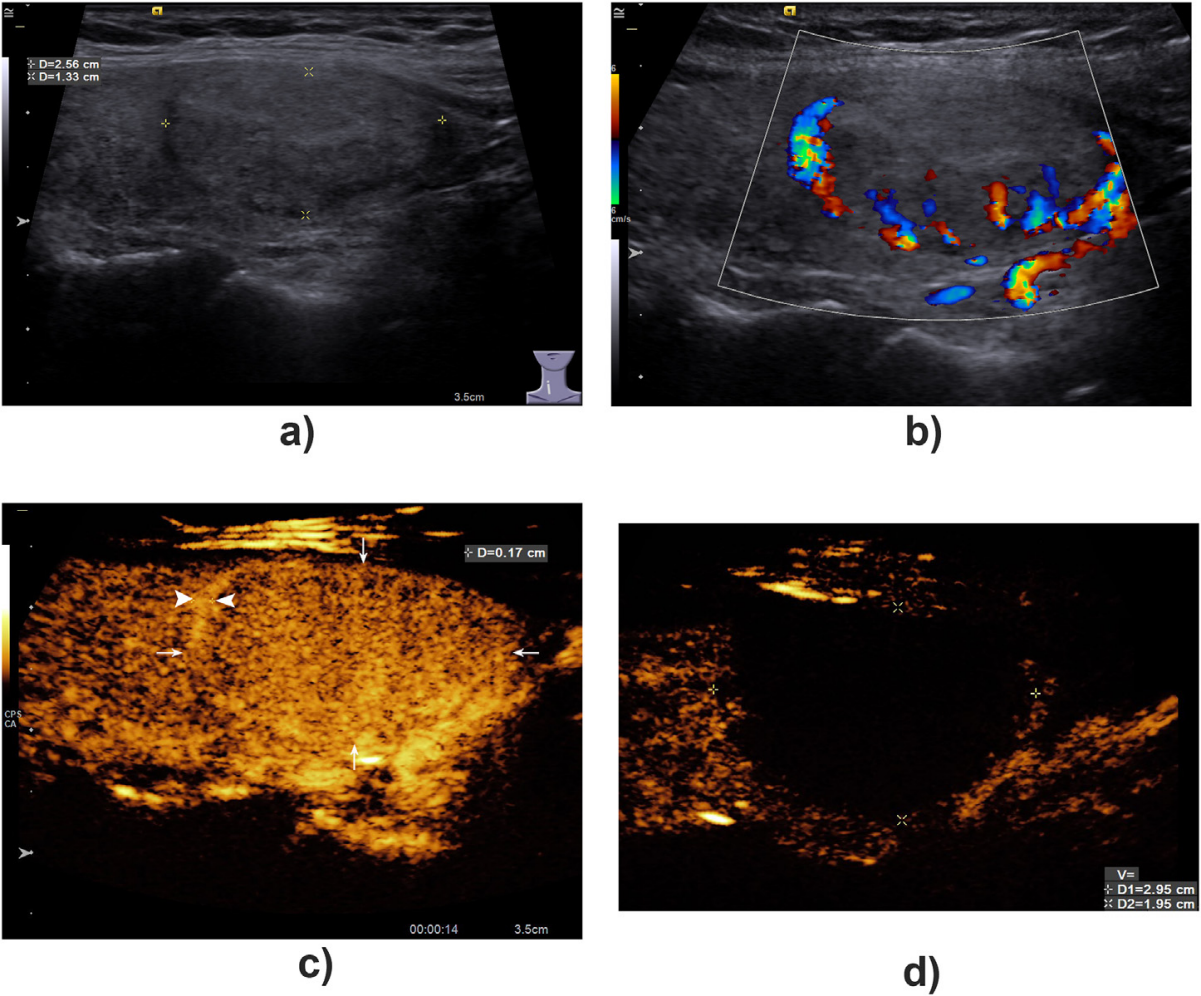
A BTN with a hyper-enhancement ring on CEUS. A 36-year-old woman with a benign nodule in her right lobe of thyroid for 8 years follow-up. The size of the nodule was 2.56 × 1.33 cm before ablation treatment. Conventional ultrasound showed that the nodule was isoechoic with a dark ring around it (A). Linear flow signals were shown around the nodule on color flow image (B). On contrast-enhanced ultrasound, the nodule showed uniform iso-enhancement (arrow), and hyper-enhanced ring (arrowhead) with a thickness of 0.17 cm could be detected around it in arterial phase (C). One month after treatment, CEUS was reexamined. It was found that the non-enhanced area was 2.95 × 1.95 cm and it could completely cover the whole lesion and its surrounding arterial ring (D).
